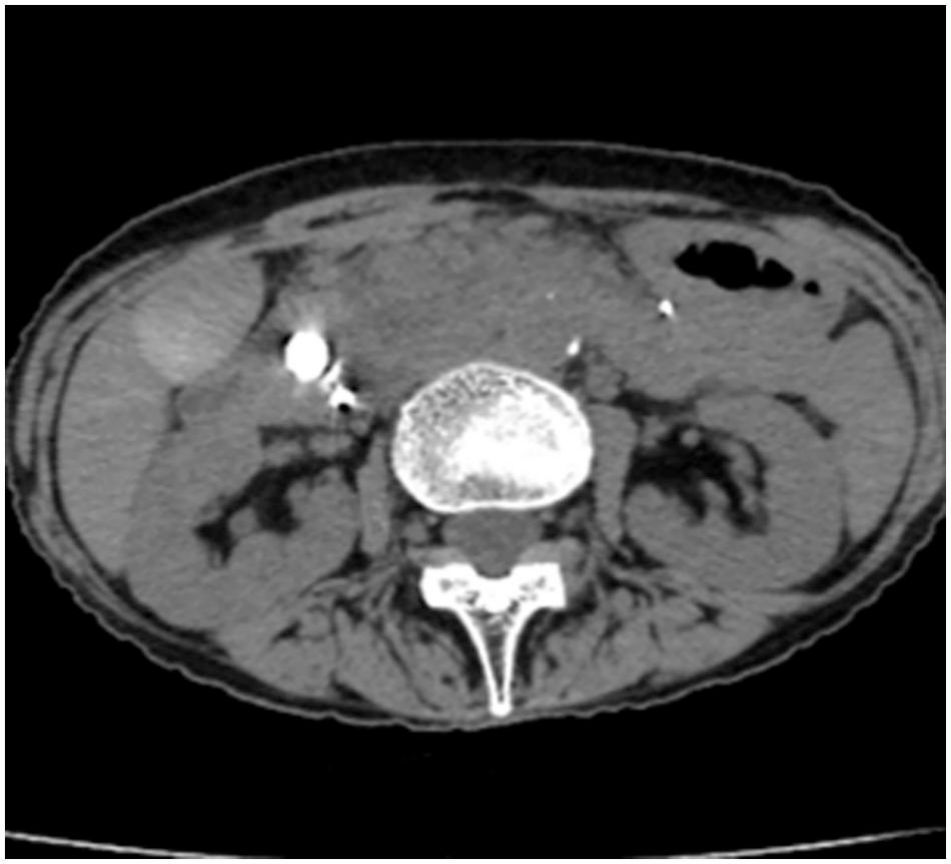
CT five days after TAE. Non-contrast-enhanced CT shows lipiodol deposition corresponding to a pseudoaneurysm in the pancreatic head.
